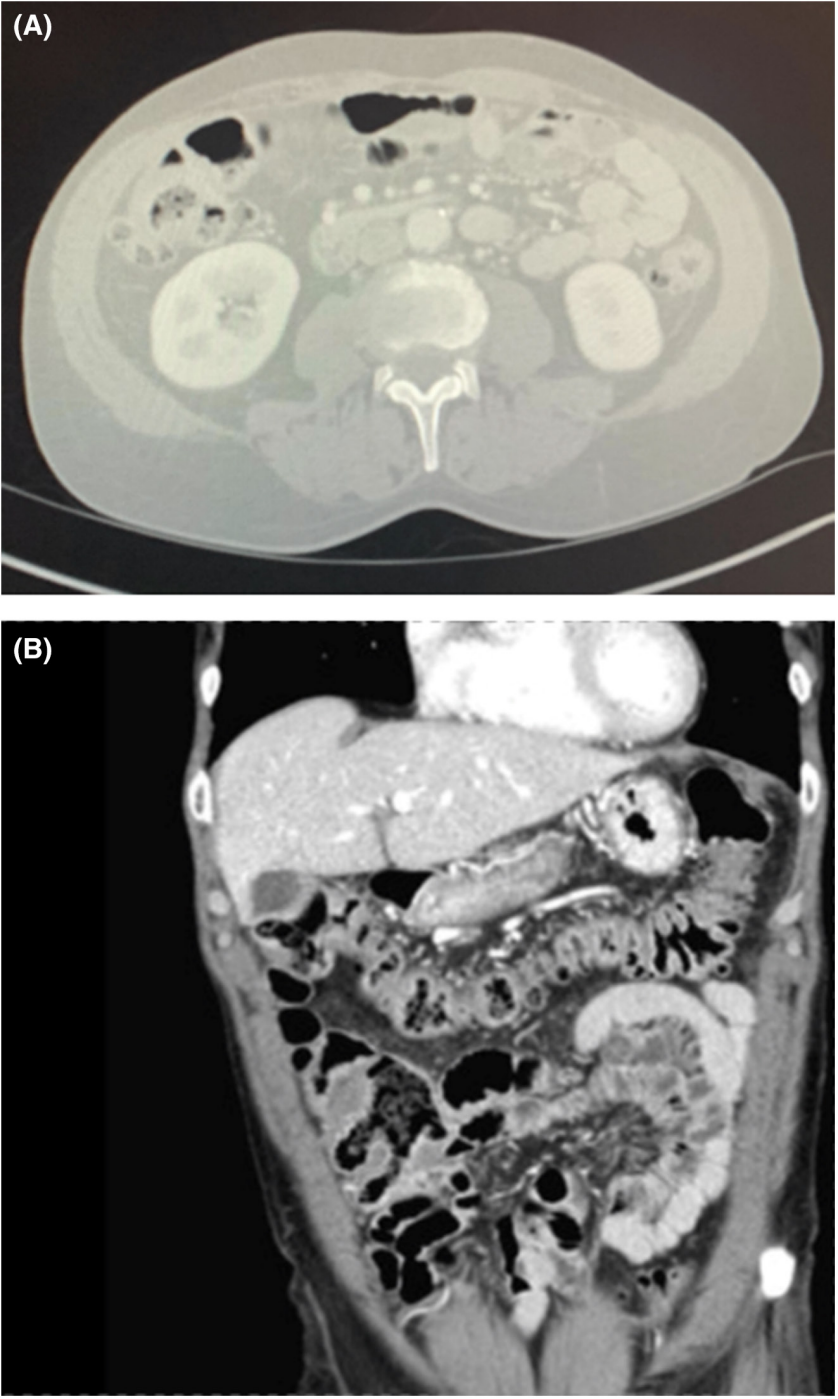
(A). Computed tomography (CT) axial view of abdomen showing nonspecific wall thickening in the cecum and proximal ascending colon. (B). Computed tomography (CT) coronal view of abdomen showing nonspecific wall thickening in the cecum and proximal ascending colon
